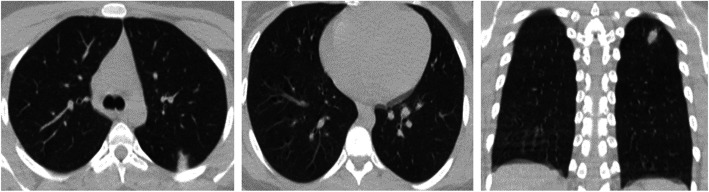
Female patient, 32 years old, was complaining of dry cough and sore throat and was confirmed to be COVID-19 by RT-PCR. These axial and coronal non- contrast CT chest images (lung window) show fairly typical findings with moderate suspicion of COVID-19 pneumonia in terms of single peripheral ground-glass opacity within the left upper lobe with CORADS-2A. Left upper lobe has score 1 (with < 5% involvement), while the right upper, middle and both lower lobes show no involvement with score 0, so total CT lung severity score is 1 with 4% lung involvement denoting minimal grade of lung affection
